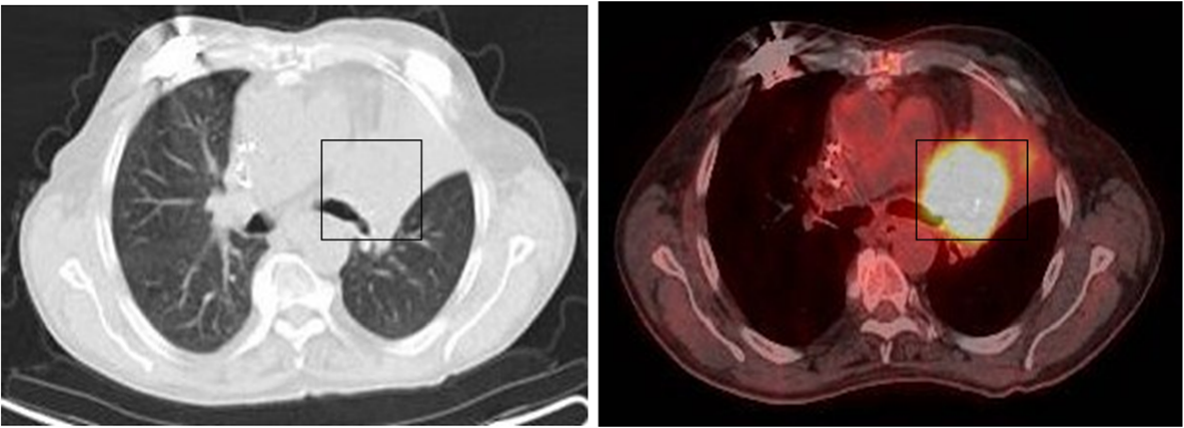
Planning-PET-CT imaging by use of “fludeoxyglucose F 18” tracer. Hypermetabolic activity of bronchial carcinoma on the left main bronchus with restriction of those.
